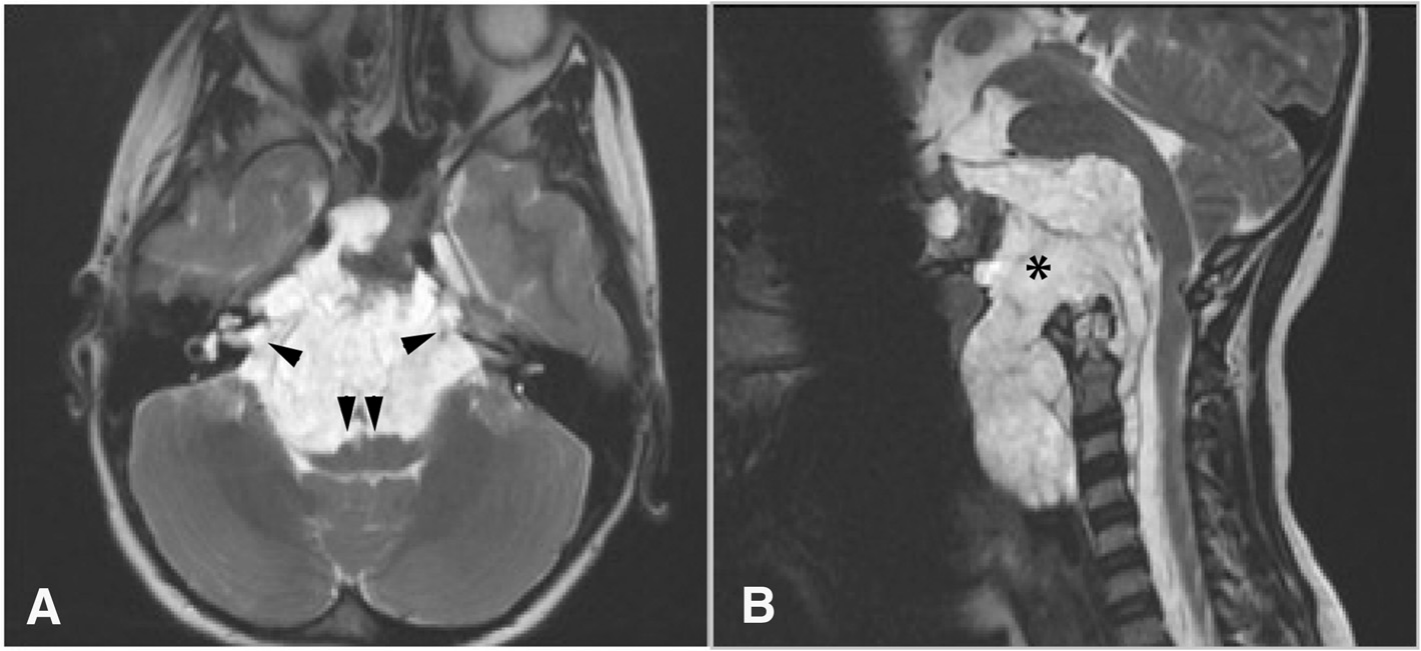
T2 weighted MRI of a clival chordoma. a. Axial plane. A voluminous chordoma developed from the clivus with extension to both cerebellopontine angles (single arrowheads). Brainstem is pushed back posteriorly (double arrowhead). b. Sagittal plane. The chordoma eroded the inferior part of the clivus (asterisk) and developed anteriorly to the cervical spine. Typical lobulated appearance with multiples hypointense septae can be observed
